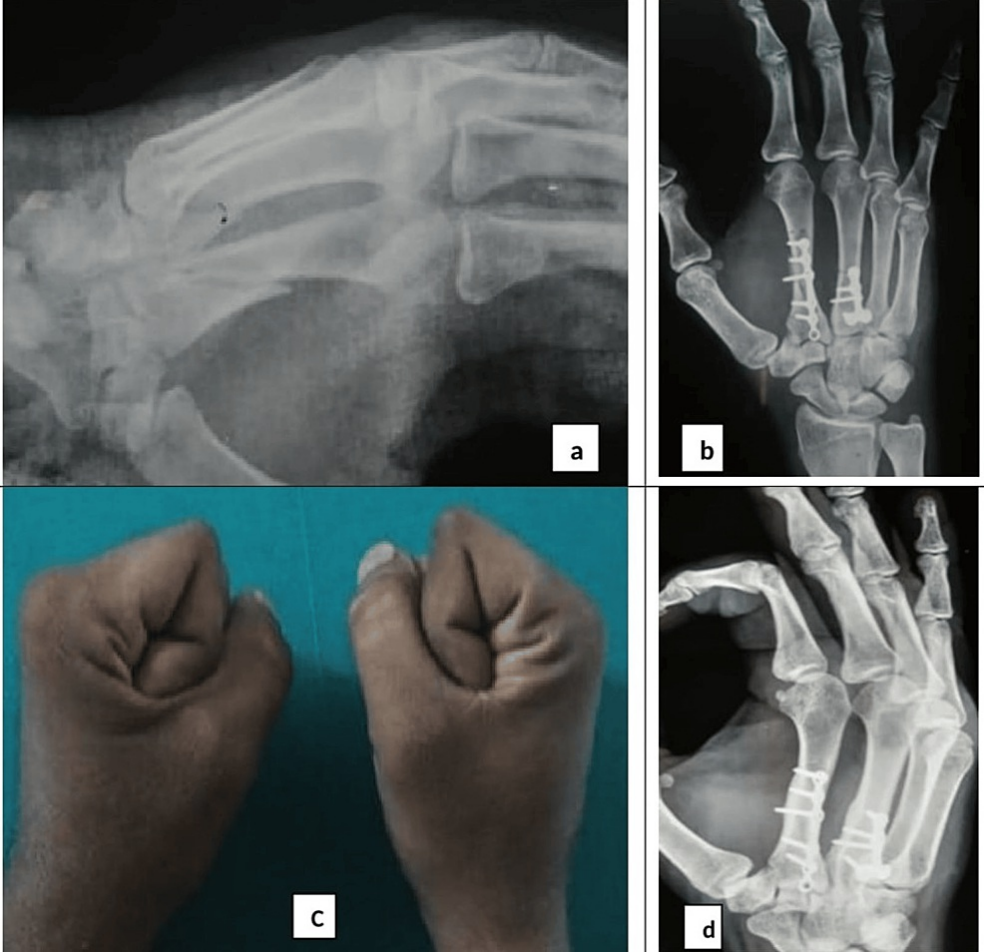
Radiographs showing oblique fracture of 2nd and 3rd Metacarpals (a), ORIF with a plate and interfragmentary screws (b), clinical picture showing comparable excellent active ROM (c), radiograph showing complete union at fracture sites (d).
